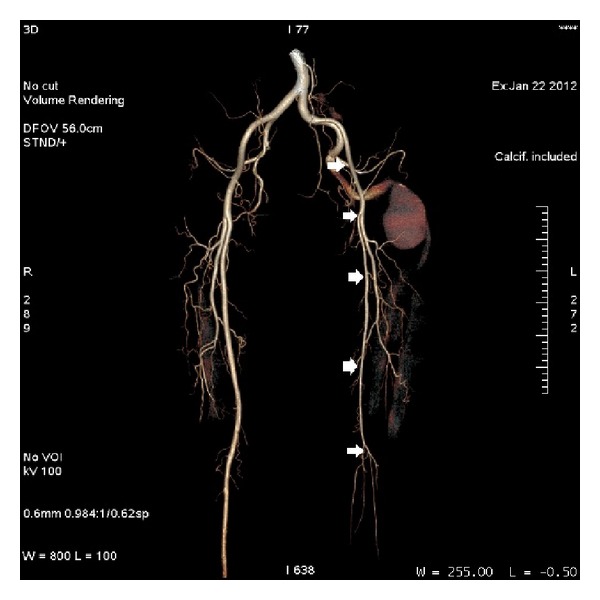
CT angiogram. The arrows depict the course of the external iliac artery and the superficial femoral system. Note that the femoral vessel is hypoplastic compared with the normal opposite limb and ends in the thigh.
